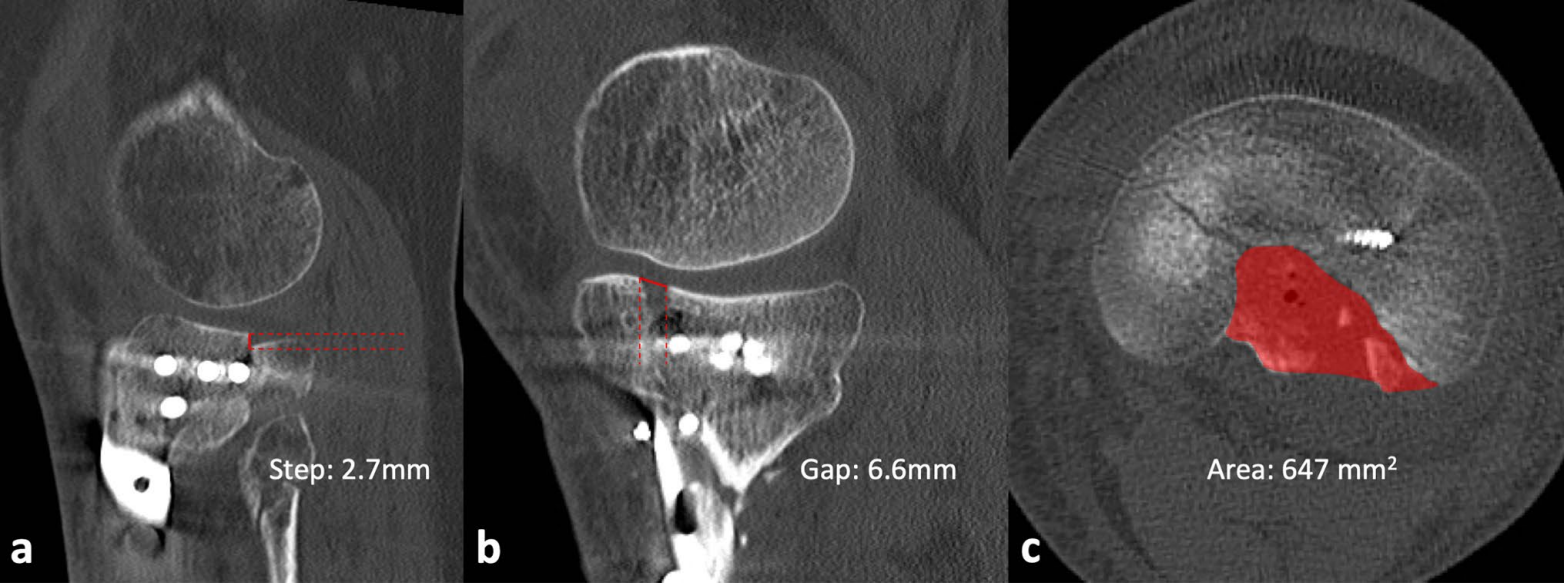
Digital measurement of steps, gaps and comminution area of the articular surface on CT scans. Examples of maximum remaining step height (a), gap size (b) and size of the comminution area of the articular surface (c) on postoperative CT scans after surgical reconstruction of the tibial plateau fractures
